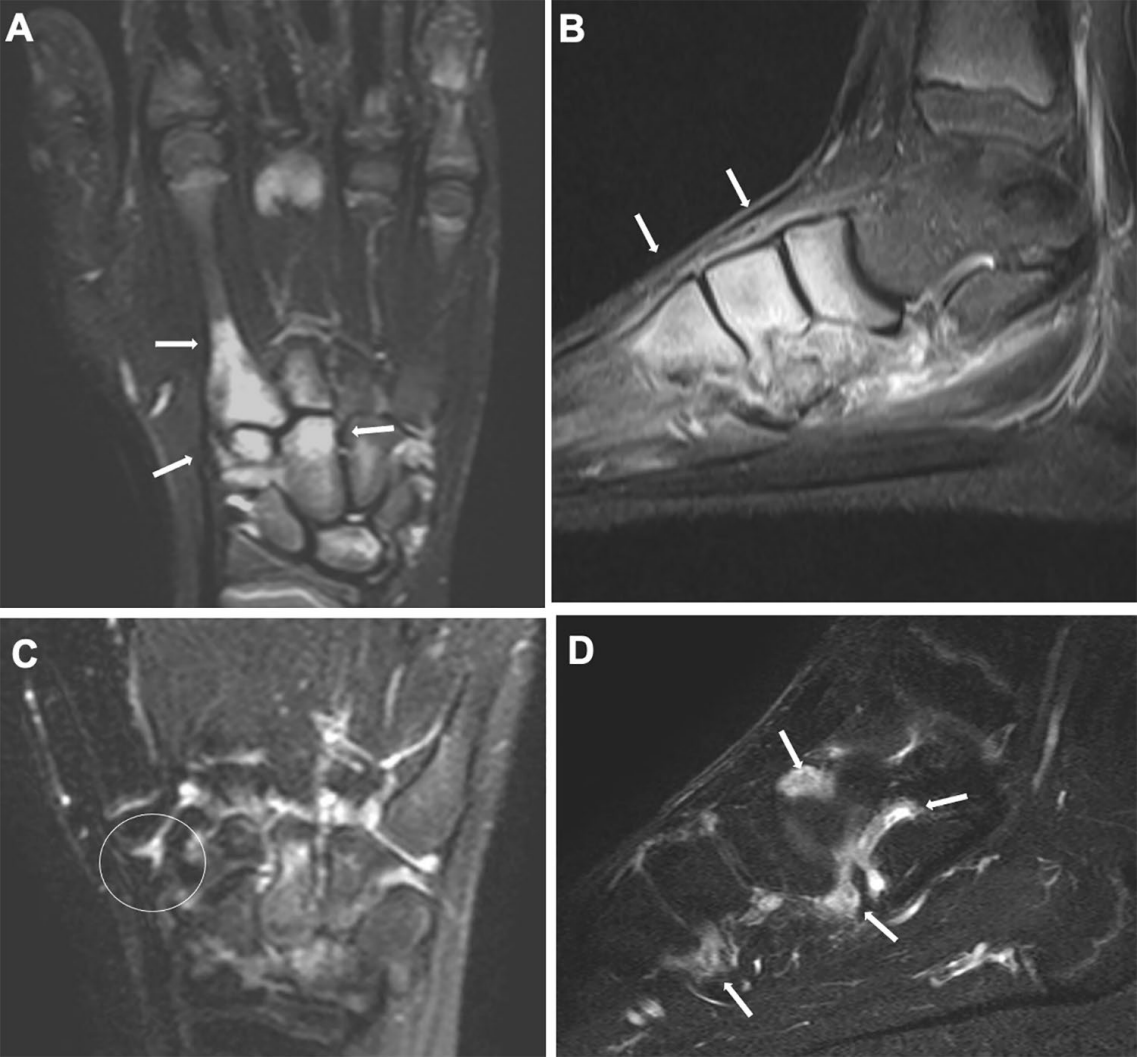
RF-positive polyarticular type JIA. A-D. An 8-year-old boy. Fat-suppressed T2-weighted coronal image of the hand (A) and sagittal image of the foot (B) showed extensive bone marrow edema of the carpals, metacarpals, tarsals, and metatarsals (arrows, A, B). After 6 months of treatment, bone marrow edema had reduced. However, carpal erosions (circle, C) and diffuse synovial thickening of the intertarsal joints (arrows, D) became manifest
